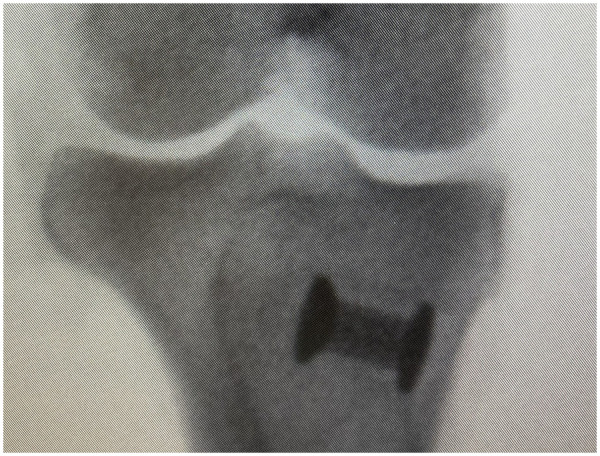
Intraoperative x-ray image.
